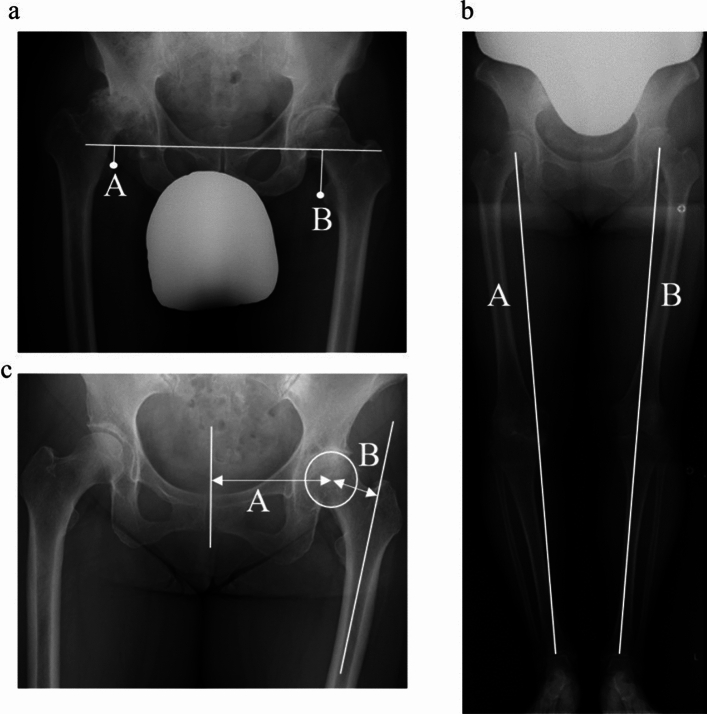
Hip parameters.a B – A = Radiographic leg length discrepancy. b B – A = Functional leg length discrepancy. c A = Acetabular offset, B = Femoral offset
